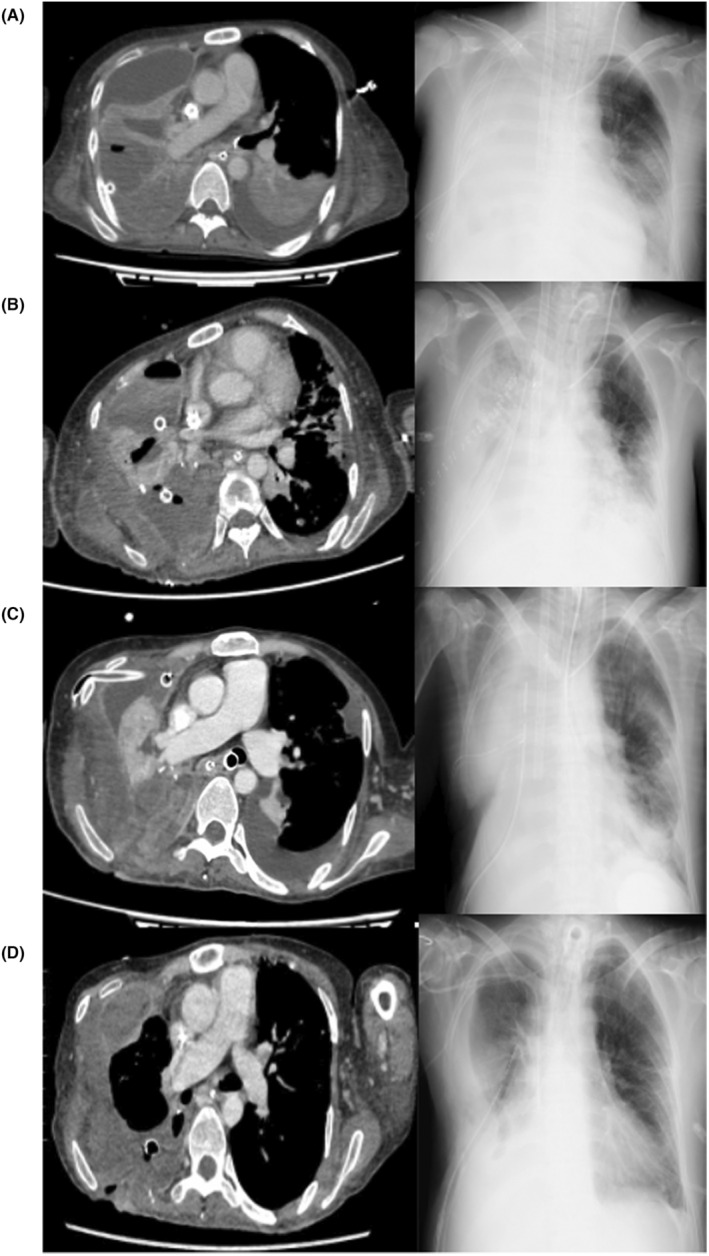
The transition of chest computed tomography (CT) and chest X‐ray. (A) Day 4. We confirmed the characteristics of the lung abscess. As intercurrent air leakage was present, we decided to perform thoracic surgery. (B) Day 17/18. Chest CT was performed on Day 17, and chest X‐ray on Day 18. On Day 17, we performed CT because the circulatory dynamics were unstable and we suspected sepsis. On the same day, re‐thoracotomy was performed. (C) Day 27. The right lung was pale in appearance. On Day 32, independent lung ventilation (ILV) was again started for the purpose of physiological lung separation. After that, transparency of the right lung gradually improved. (D) Day 56. Veno‐venous extracorporeal membrane oxygenation was decannulated on Day 48. Transparency of the right lung improved.
